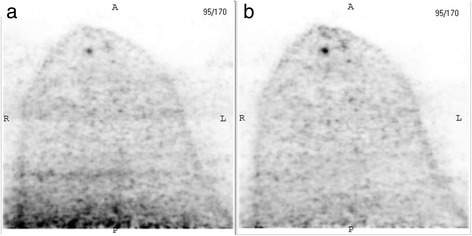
Transaxial images from a patient study demonstrating a small papilloma in the anterior portion of the breast. Cross-talk (grade 1) from non-breast F-18 FDG activity into the breast with the original reconstruction algorithm (a) is significantly reduced with the updated algorithm (b)
